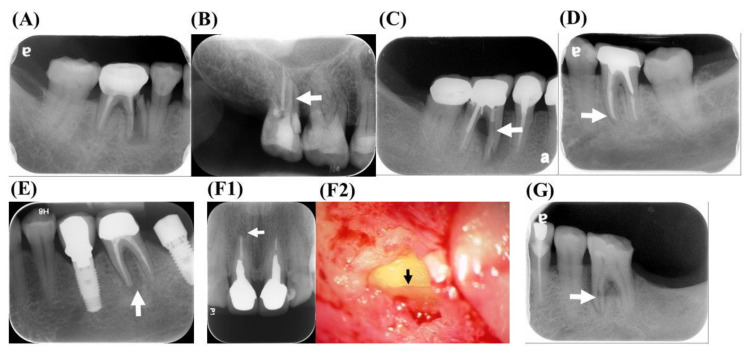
Radiographic features of the VRFs. (A) Displacement of fractured root fragment, (B) radiolucent lines within the root canal (white arrow), (C) unusual radiolucent space between the canal wall and intracanal material (white arrow), (D) widening of the periodontal ligament (PDL) space (white arrow), (E) periradicular radiolucent halo (white arrow), (F1) root resorptions corresponding to the fracture line (white arrow); (F2) VRF was further diagnosed via exploratory surgery (black arrow) and (G) a widening of the root canal space (white arrow).
